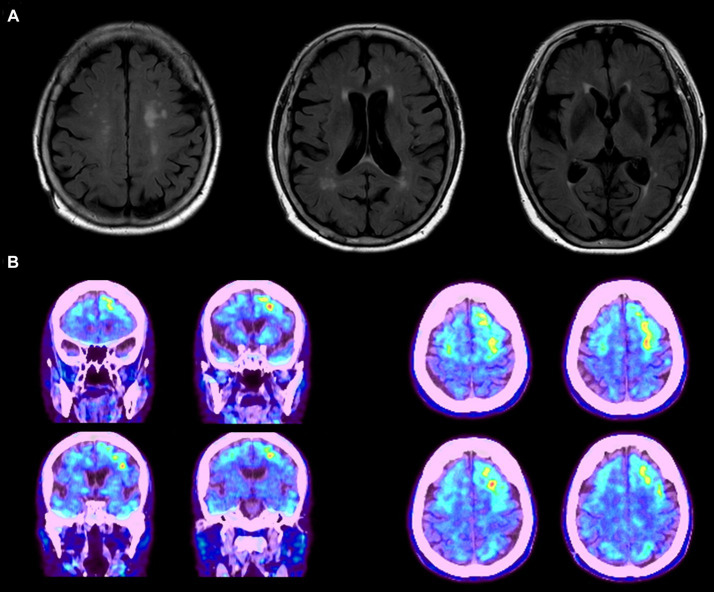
(A) MRI data of the proband patient displaying diffuse cortical atrophy and enlarged ventricles. (B) Abnormal amyloid deposits observed in the frontal, temporal, and occipital lobes, with predominant accumulation on the left side. The images represent data from a single patient as an example.
